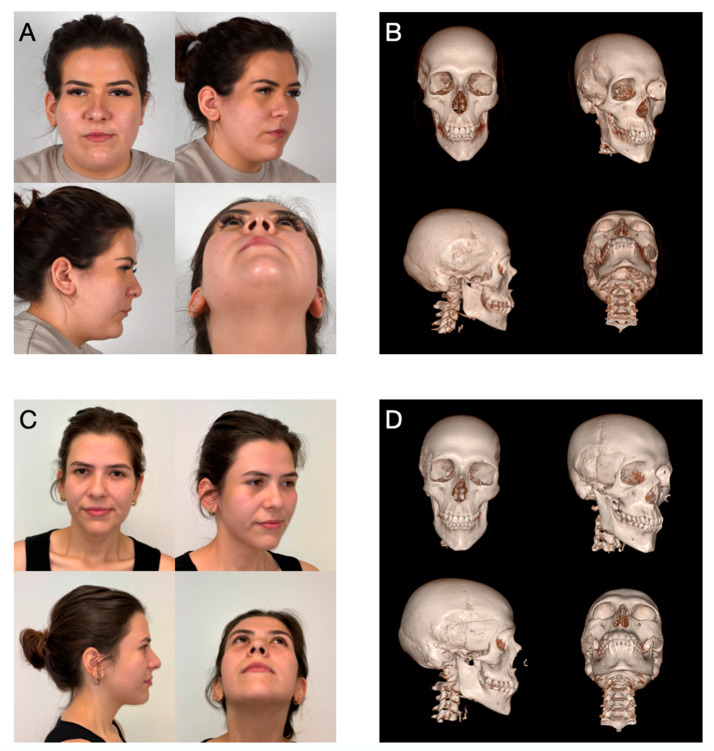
Clinical and computed tomography status before and after the procedure: Preoperatively, both clinically on 24 February 2021 (A) and radiologically on 8 March 2024 (B), a pronounced deformity in the area of the right temple is evident. Postoperatively, a clinical examination on 15 July 2024 (C) shows a homogeneous and symmetrical alignment of the facial contours. The radiological follow-up on 10 July 2024 (D) confirms the correct positioning of the PSI.
